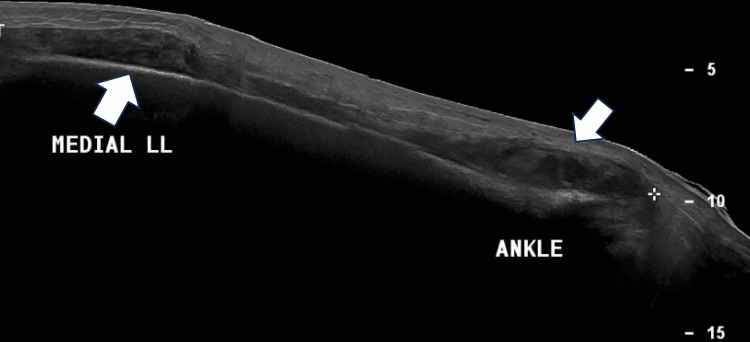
Ultrasound image of the right lower limb (LL) The image showed a deep-seated collection (white arrows) extending from the ankle to the proximal leg
